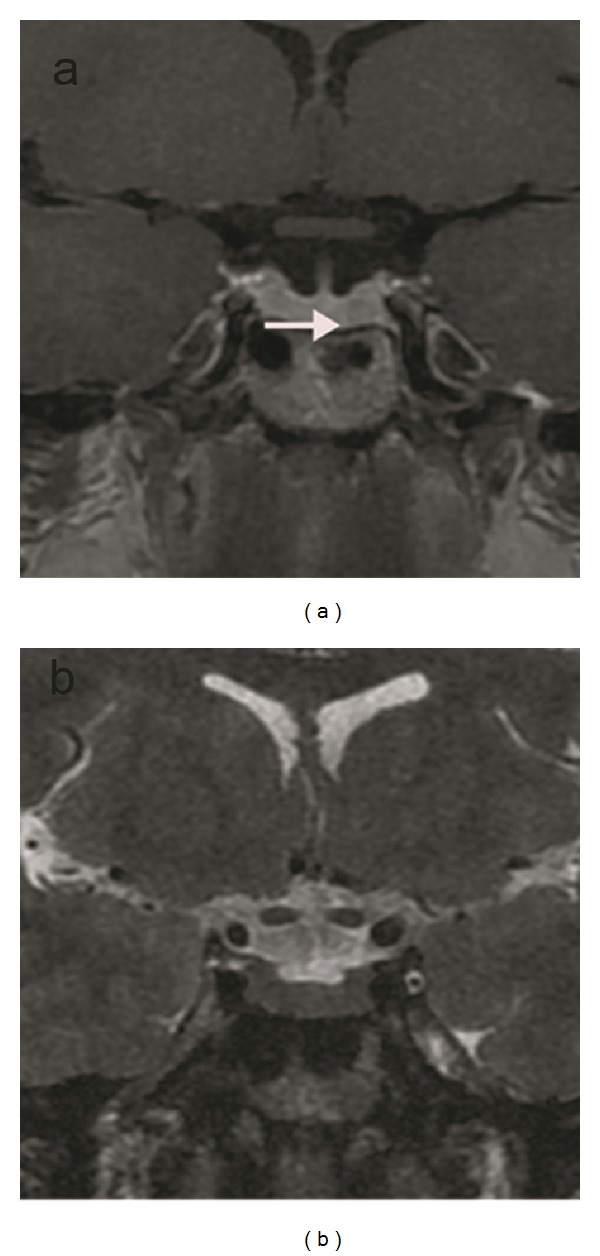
A microadenoma with hyperintensity on the T1-weighted coronal image enhanced with IV-GBCM on left side of the gland is shown (a) (arrow). T2-weighted coronal image (b) shows no abnormalities.
